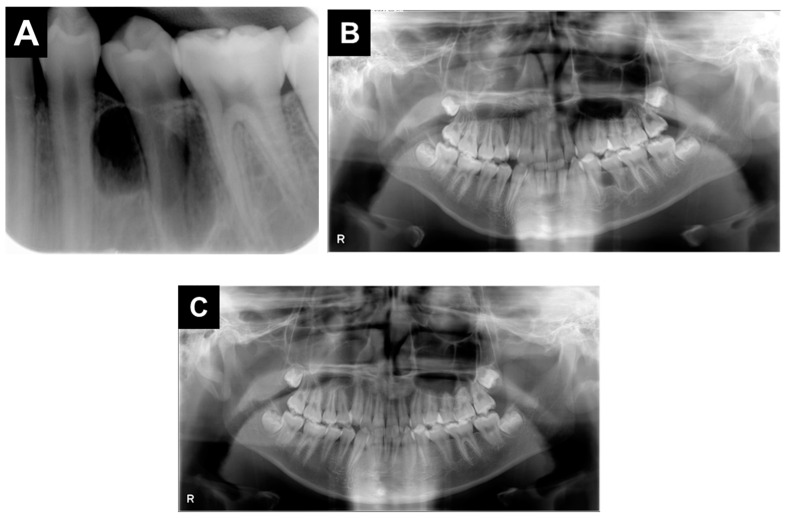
(A) Periapical and (B) panoramic radiography images after surgery obtained at follow-up examination. (C) Panoramic radiography image at 1-year follow-up.
